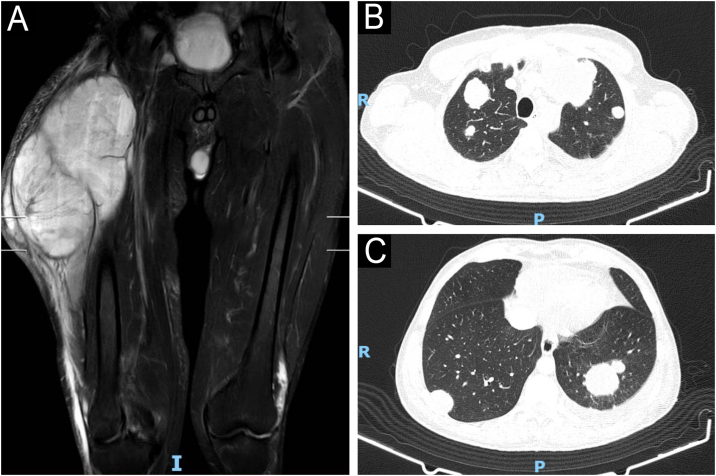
(A) Pre-surgery MRI of the thigh showing an expansile mass in the region of the extensor muscles measuring approximately 23.3 by 12.8 × 13.0 cm, with a volume of approximately 2000 mL, with a heterogeneous liquid content, areas suggestive of hemorrhage, and peripheral contrast uptake. (B and C) Contrast-enhanced chest computed tomography performed 68 days after complete excision of the lesion showed several pulmonary nodules with a neoplastic appearance.
